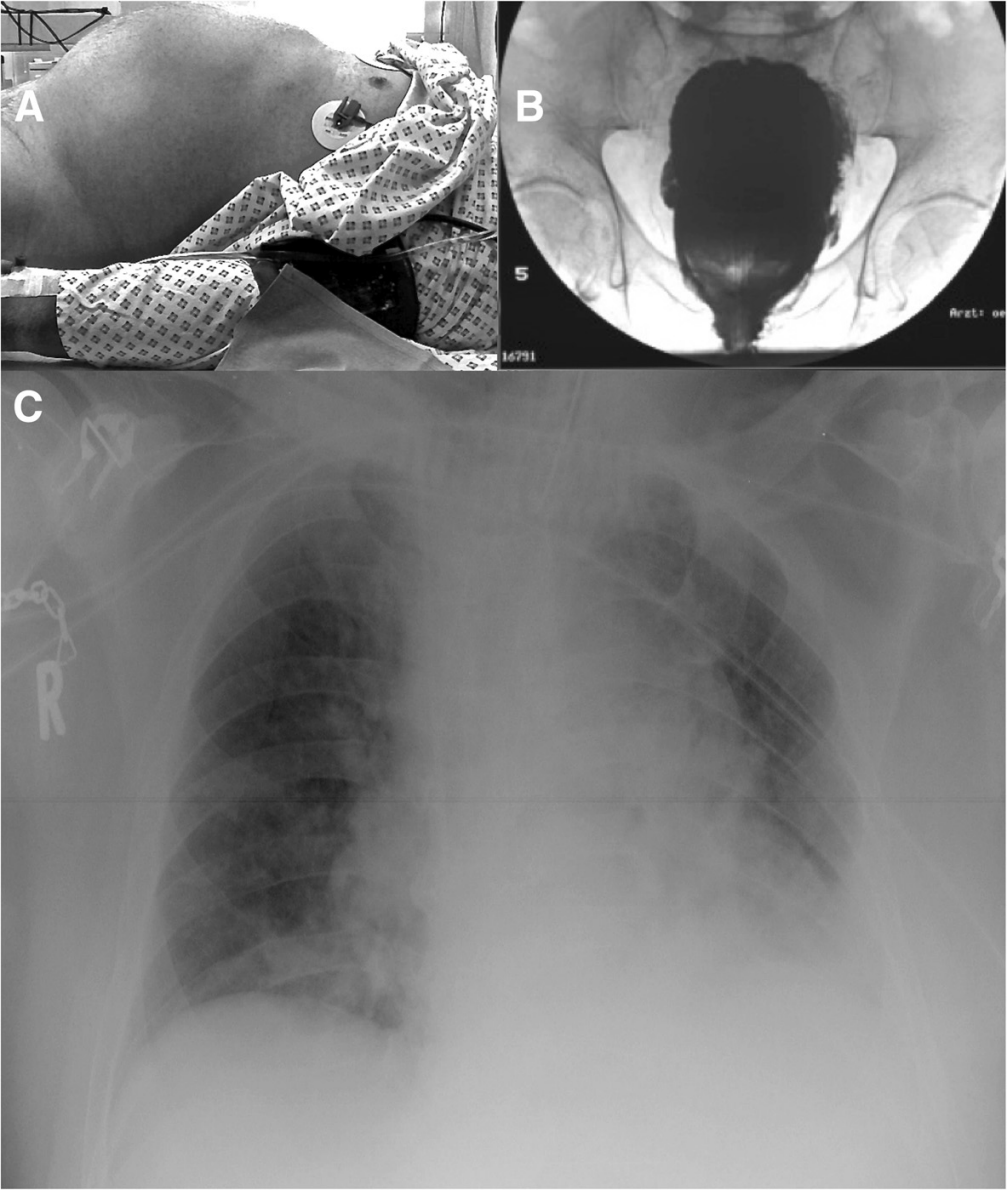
Patient images. A) Patient on the x-ray table. Please note the prominent abdominal region. Measured intra-abdominal pressure was 26 mmHg. B) Contrast agent x-ray of the bladder. There are no signs of bladder perforation. C) X-ray of the patient’s thorax during ventilation. Endotracheal tube and central venous line were in the correct position. There were signs of atelectasis of the left lower lung and cephalic displacement of the diaphragm due to the intra-abdominal volume augmentation and despite the restricted conditions for the x-ray in the ICU bed.
